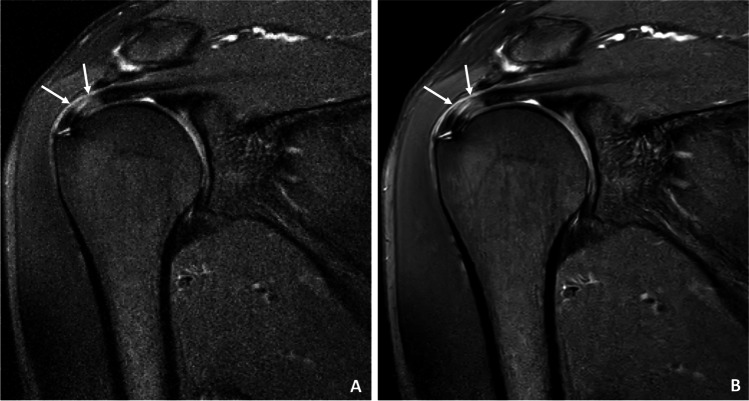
A 54-year-old patient with tendinopathic changes of the rotator cuff, in particular the supraspinatus tendon. A Standard coronal T1-weighted sequence with TSE. B CS DL reconstruction of the T1-weighted TSE sequence with overall reduced noise and smoother discrimination of the tendinopathy of the supraspinatus tendon (white arrows)
